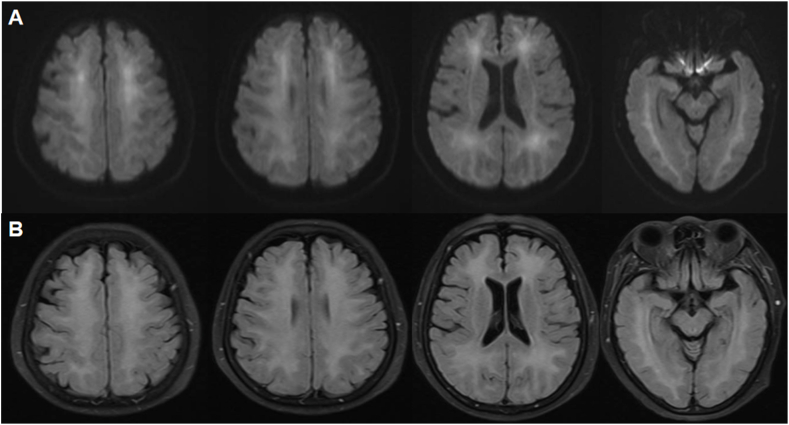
MRI findings at the presence of choreic movements 15 days after hypoxic events. DWI(A) and FLAIR (B) sequences revealed symmetrical diffuse hyperintense signals predominantly in central white matter of bilateral frontal, temporal, parieto-occipital lobes. MRI = magnetic resonance imaging; DWI = diffusion weighted imaging; FLAIR = fluid-attenuated inversion recovery.
